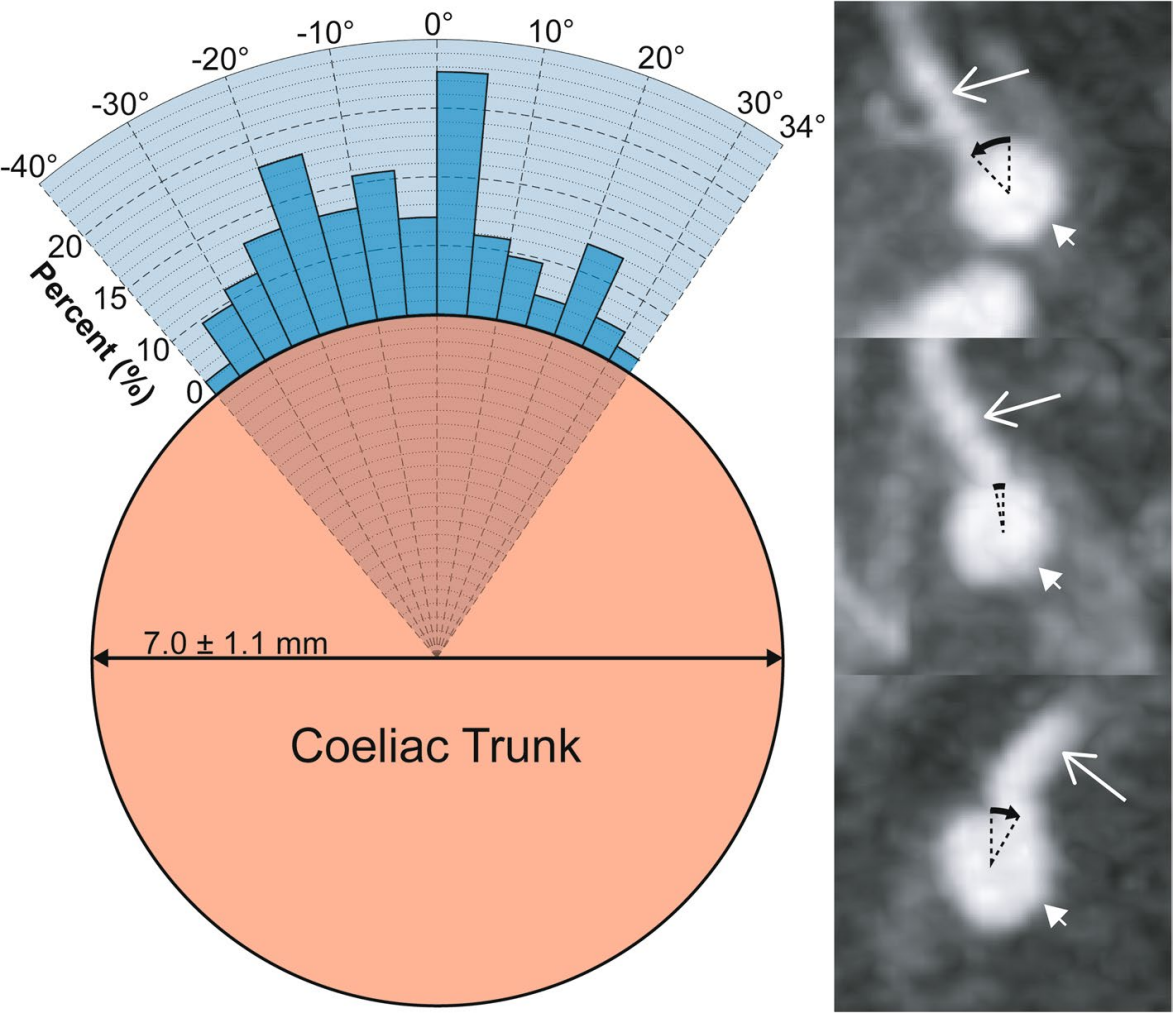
Clockwise angle of the origin of the LGA from the coeliac trunk in the para-coronal plane. Histogram of the clockwise angle of origin of LGA in a para-coronal plane through the coeliac trunk plotted as a percentage of participants. The minimum and maximum angle is represented by the radial range of the graph (− 40 to + 34°). The cross-sectional dimensions of the coeliac trunk are also included. Inset CT images show examples and calculations for the LGA clockwise angle from participants in the (top to bottom) lowest, median and highest decile. Long white arrow, LGA; short white arrow, coeliac trunk; black arrow, measured angle
